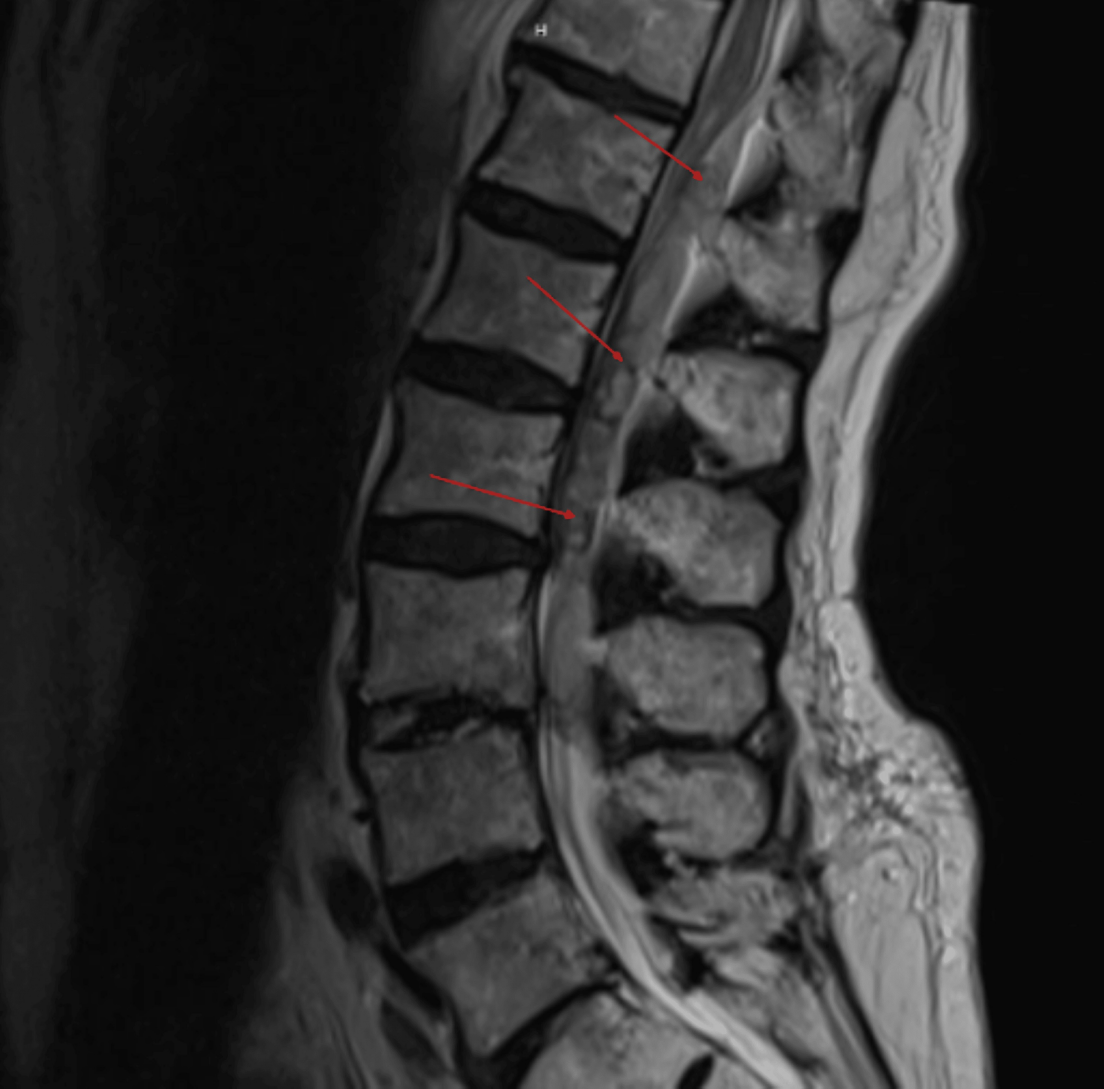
T2 sagittal MRI demonstrates hyperintensity with hypointense foci throughout. MRI: magnetic resonance imaging
